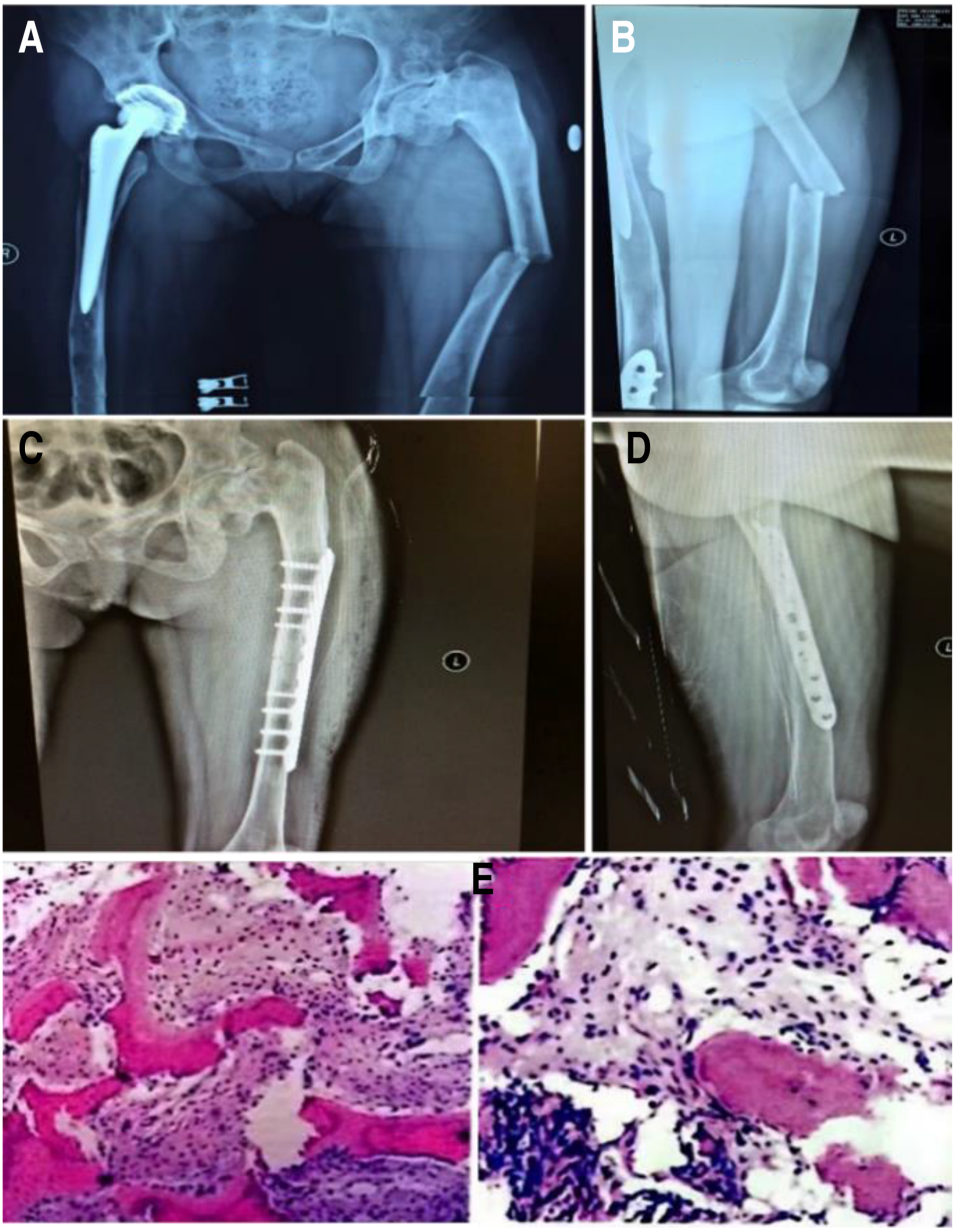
Left femoral shaft fracture under slight external force. (A,B) Preoperative x-rays, (C,D) postoperative x-rays, (E) postoperative pathology.
